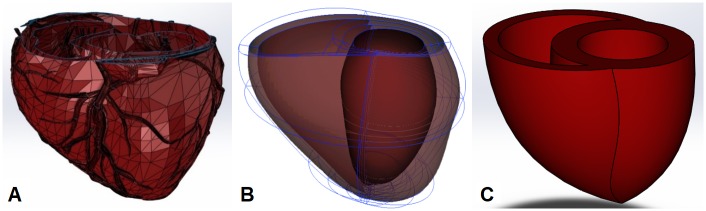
Human biventricular models. Examples of human biventricular models reconstructed from a CT scan (A); an echocardiogram scan (B); and a computer rendering of a passive human biventricular model (C) designed with these reported data.
